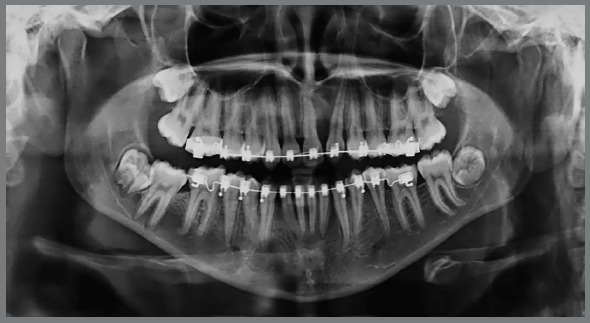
Panoramic radiograph during treatment progression, showing lack of space for eruption of the mandibular right second molar.
